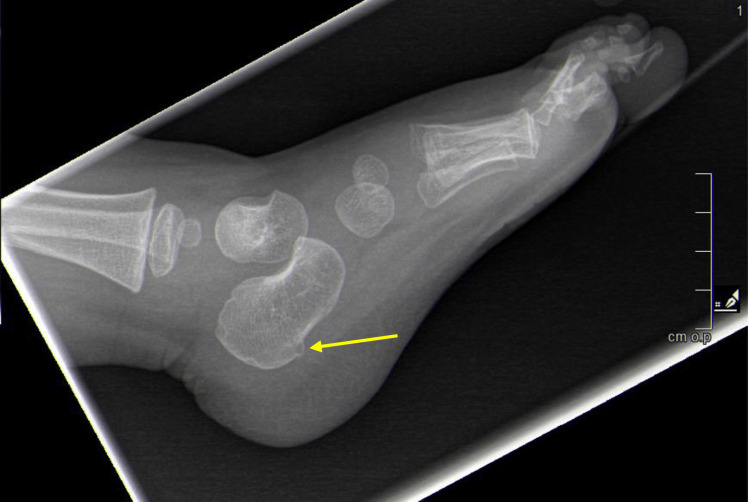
Lateral radiograph of the left foot demonstrating a toddler’s fracture. Patient history notable for limping of three weeks duration. Fracture is located at the plantar aspect of the calcaneus. Note buckle of plantar cortex and subtle periosteal reaction.
